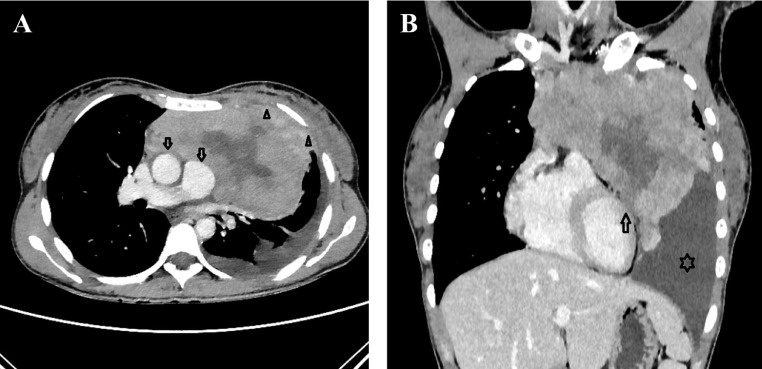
Chest computed tomography with intravenous contrast during the venous phase. (A) The axial mediastinal window shows that the anterior mediastinum contains a heterogeneously enhancing irregular mass. The mass has invaded mediastinal fat and adhered to adjacent vessels such as the aorta and pulmonary artery (arrow). The mass also adhered to the left upper hemithorax wall (open arrow). (B) The coronal mediastinal window shows the infiltration of the mass to the pericardium and epicardial fat (arrow). Also, note the presence of the left pleural effusion (asterisk).
